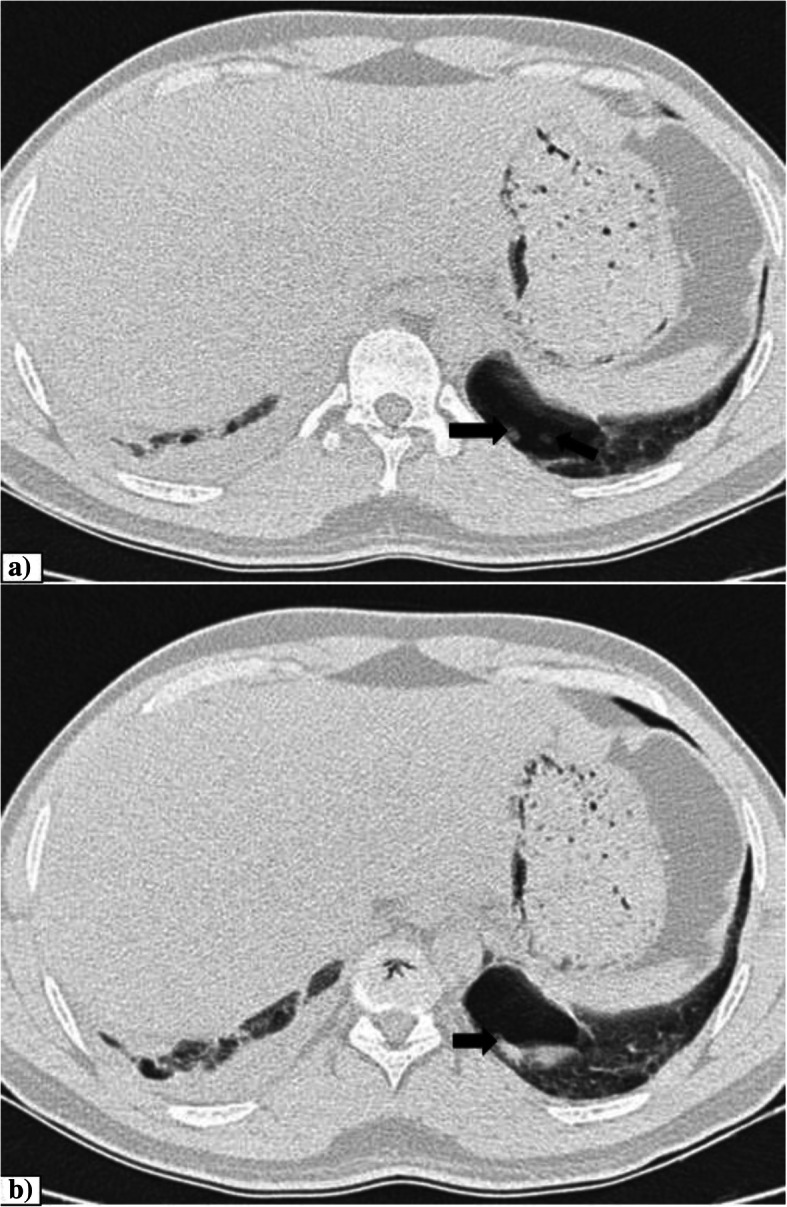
Pulmonary cavity with clots. a, b Contiguous sections of axial CT thorax of a 35-years-old COVID pneumonia patient who presented with hemoptysis, showing thin wall cavity in the posterior basal segment of left lobe with soft tissue component of hemorrhagic density as indicated by the arrows. Right pleural effusion is also seen
